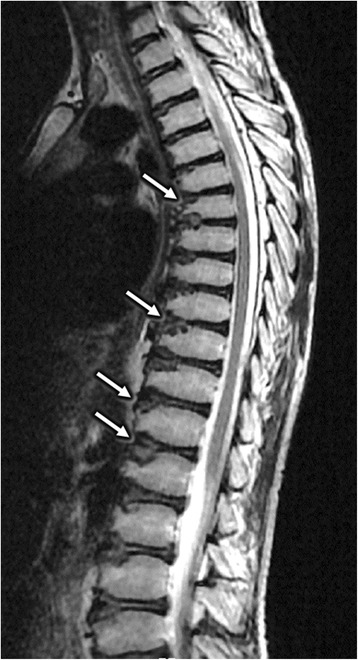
The thoracic MRI showed the dysplasia of thoracic vertebrae, which had bullet-like shapes with focal cartilage defects at the front edge (*Arrows*)
